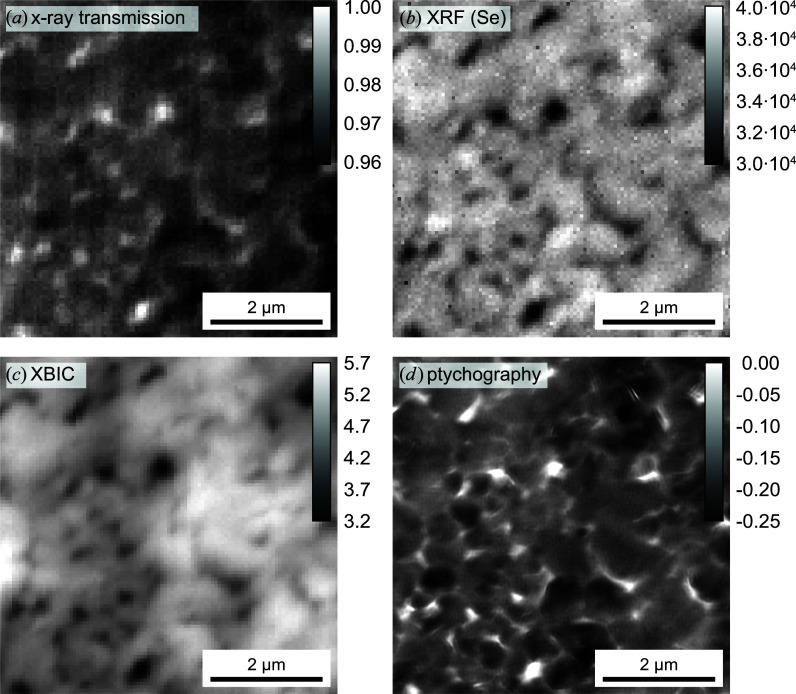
Multi-modal X-ray imaging of a solar cell with a CuIn_1−*x*_Ga_*x*_Se_2_ absorber layer. 2D maps of (*a*) the X-ray transmission signal (maximum intensity scaled to 1), (*b*) the XRF signal (sum of *K*α and *K*β) of selenium (counts per second), (*c*) the XBIC signal (nanoamperes) and (*d*) the ptychographically reconstructed phase of the object transmission function (radians). See also the greyscale bars in each image.
